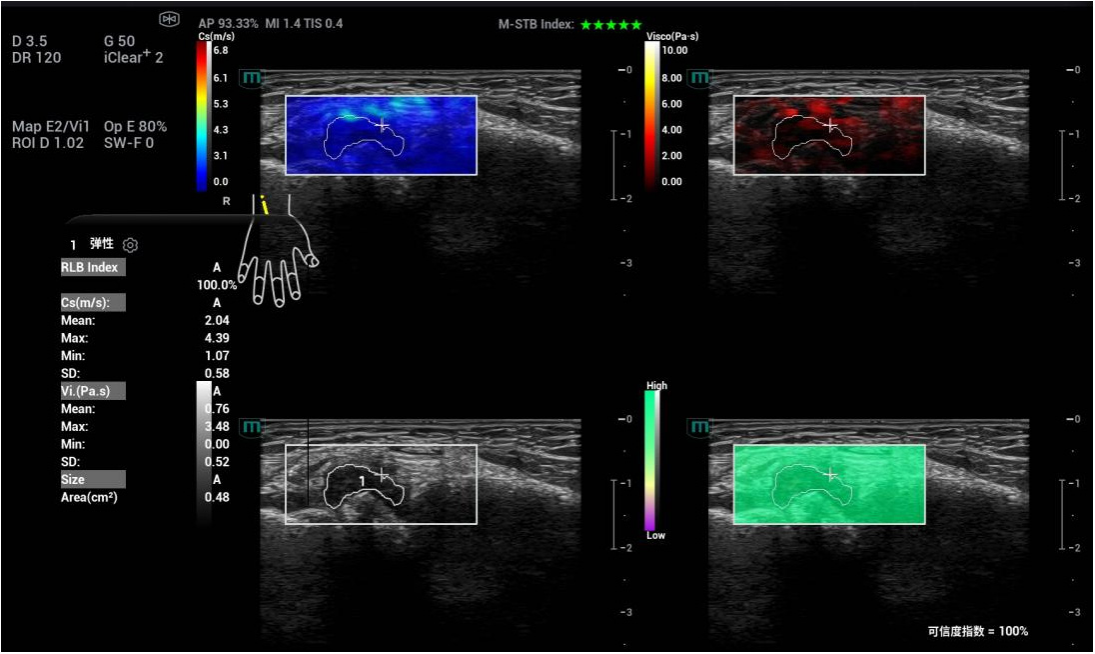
Ultrasound viscoelastic image of the left elbow joint in a patient with acute gout (AG). A 69-year-old male patient. Ultrasound viscoelastic imaging showed that the thickened synovium of the left elbow joint had a Cmean of 2.98 m/s (>cut-off value of 2.36 m/s) and a Dmean of 8.47 (m/s)/kHz [>cutoff value of 4.35 (m/s)/kHz].
